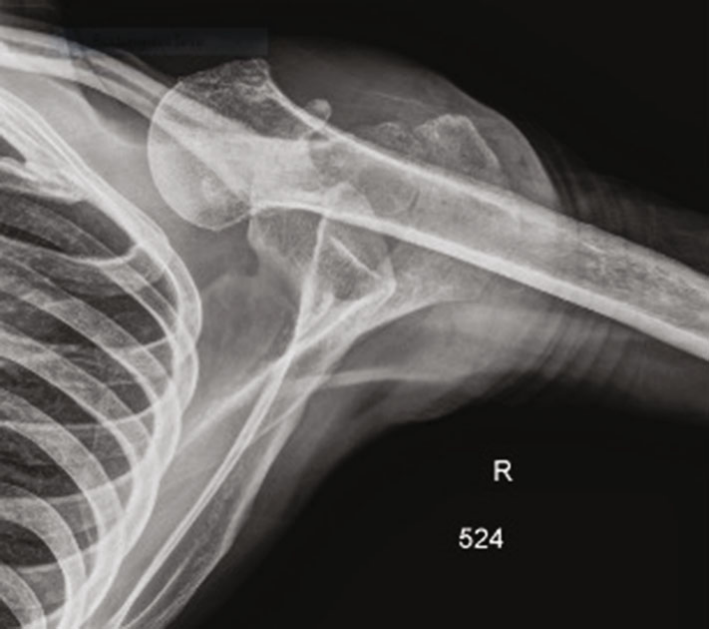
Pre-reduction axillary radiograph of the right shoulder showing anterior dislocation with medial migration of the humeral head relative to the glenoid. No associated fracture is seen.
